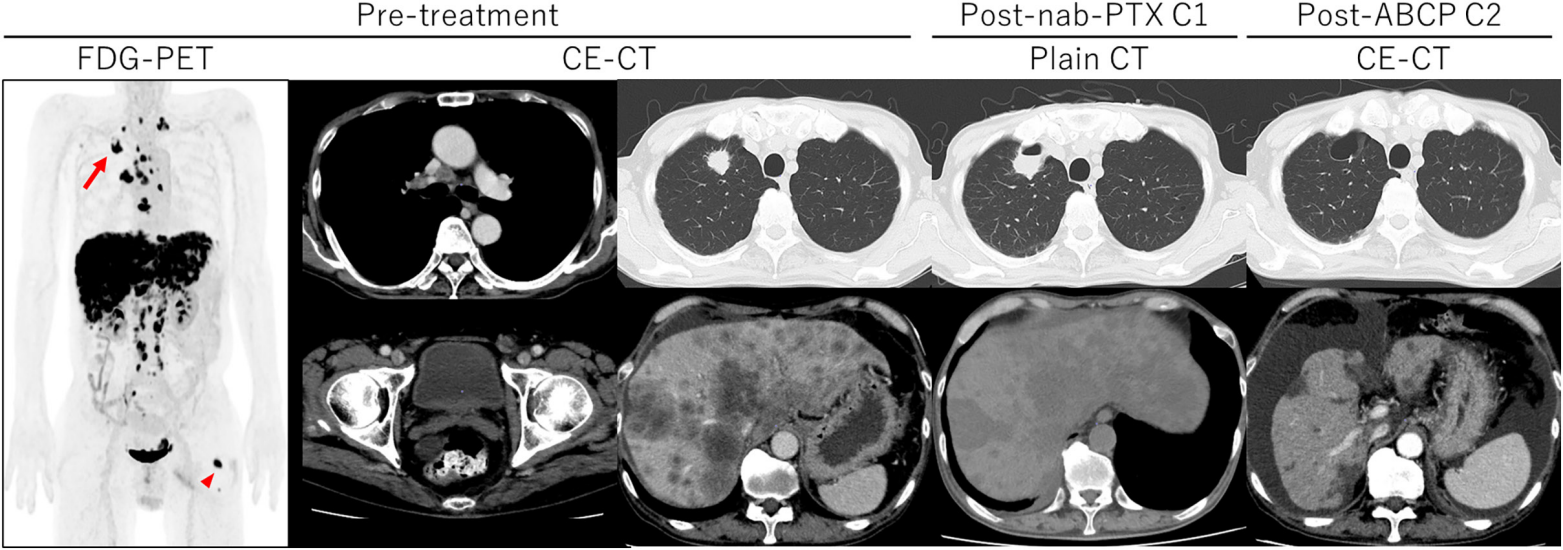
Imaging studies. Pre‐treatment 18F‐FDG‐PET revealed a primary tumour in the right upper lobe (arrow), and extensive liver and nodal metastases. The arrowhead marks a left inguinal lymph node which was subsequently biopsied. After two cycles of ABCP therapy, the primary lung lesion became undetectable, whereas ascites increased.
